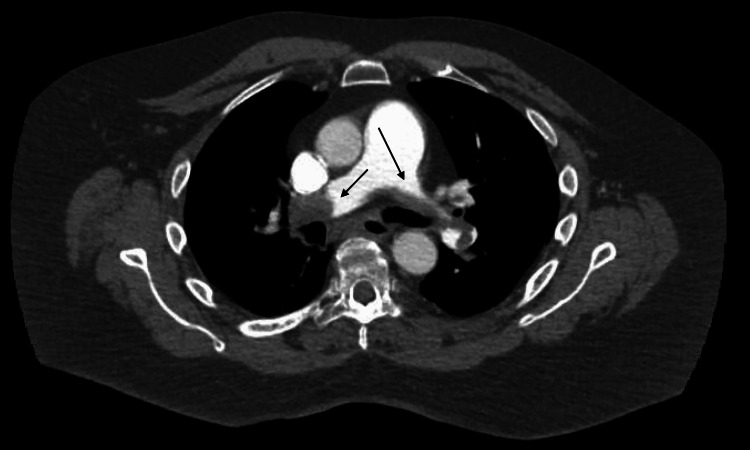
CTA axial view of chest demonstrating saddle pulmonary embolism with a large clot burden Black arrows point to the thromboembolisms in the pulmonary artery. CTA, computed tomography angiography.
